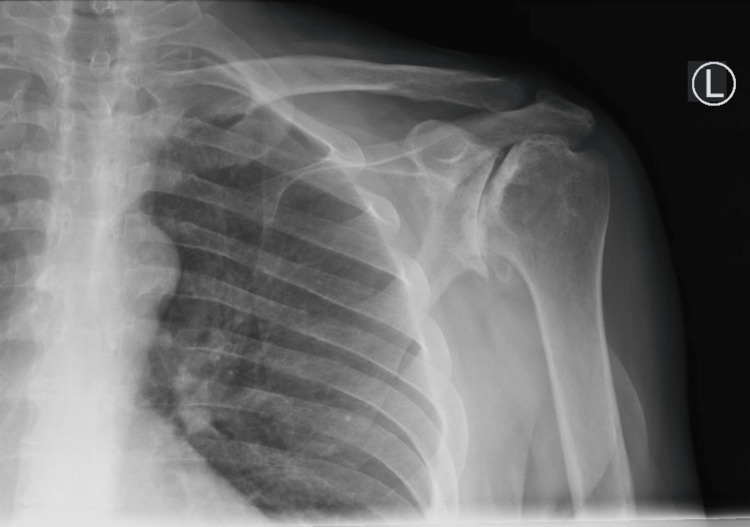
Preoperative X-rays of the left shoulder The image depicts a Grashey view of the left shoulder
